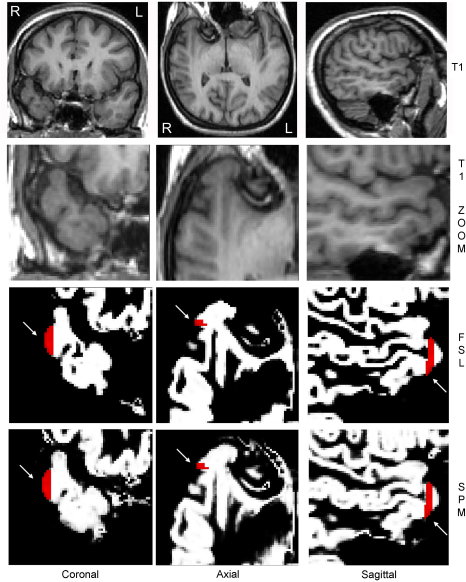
Top panels show full brain and zoomed T1-weighted volume images of right temporal lobe in patient 8, oblique coronal, axial and sagittal views. The third row shows grey matter probability maps (created using FSL). The fourth row shows grey matter class images (created using SPM5) of the right temporal lobe, same views as the zoomed T1-weighted images above. In the third and fourth rows the ROI (red) in the middle temporal gyrus is overlaid on the segmented images and is in the correct location (arrows). (For interpretation of the references to colour in this figure legend, the reader is referred to the web version of the article.)
